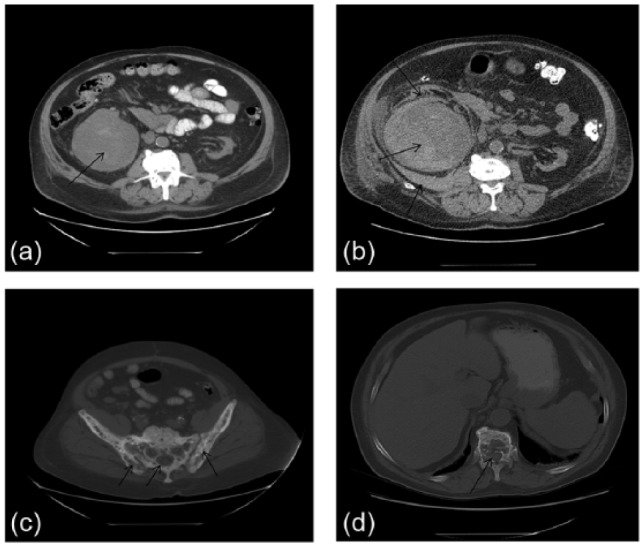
Computed tomography of the abdomen. Images “a,” “c,” and “d” are taken from a study performed on the day prior to admission. Image “b,” which approximates the tomogram in image “a,” is taken from a study done on the fourth hospital day. Image “a” shows a large, complex mass in the right kidney (arrow). Image “c” demonstrates skeletal changes of Paget’s disease in the ilia and sacrum (arrows). Image “d” shows bone lysis in the T12 vertebral body and violation of the cortex adjacent to the spinal canal (arrow). In image “b,” blood has accumulated anterior to, within, and posterior to the mass (arrows).
